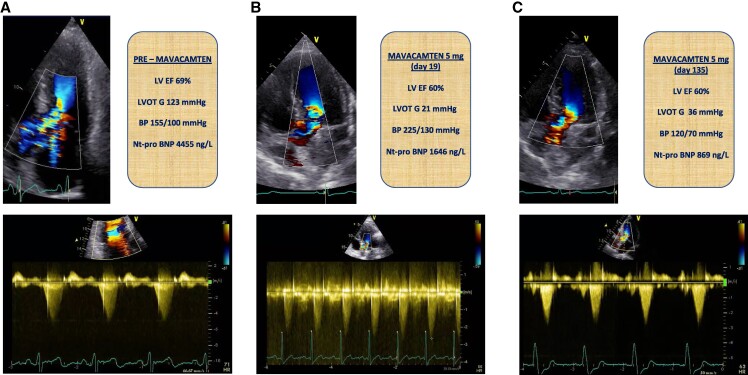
Serial echocardiographic evaluation pre mavacamten introduction, at 19 days of treatment and at 135 days. (*A*) shows bottom left apical view 5 chamber showing the sub-aortic acceleration with the associated mitral insufficiency, whereas below the continuous Doppler spectrum on the left ventricular outflow tract is showed, up to 123 mmHg. (*B*) presents bottom left apical view 5 chamber showing the reduction sub-aortic acceleration, whereas below the continuous Doppler spectrum on the left ventricular outflow tract shows no significant gradient at 21 mmHg. (*C*) shows bottom left apical view 5 chamber confirming the reduction of sub-aortic acceleration, and below the continuous Doppler spectrum on the left ventricular outflow tract shows sustained reduction of the gradient, at 36 mmHg. BP, blood pressure; LVEF, left ventricular ejection fraction; LVOTG, left ventricular outflow tract gradient.
